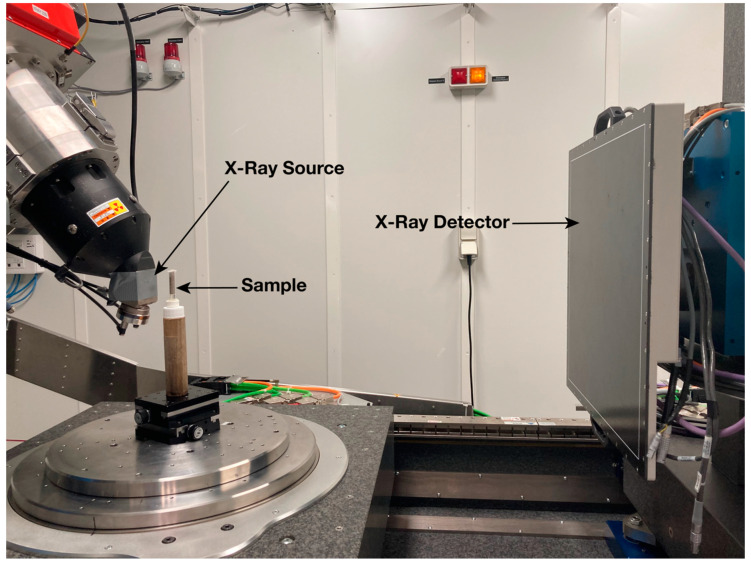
Picture of the X-ray microtomograph setup.
